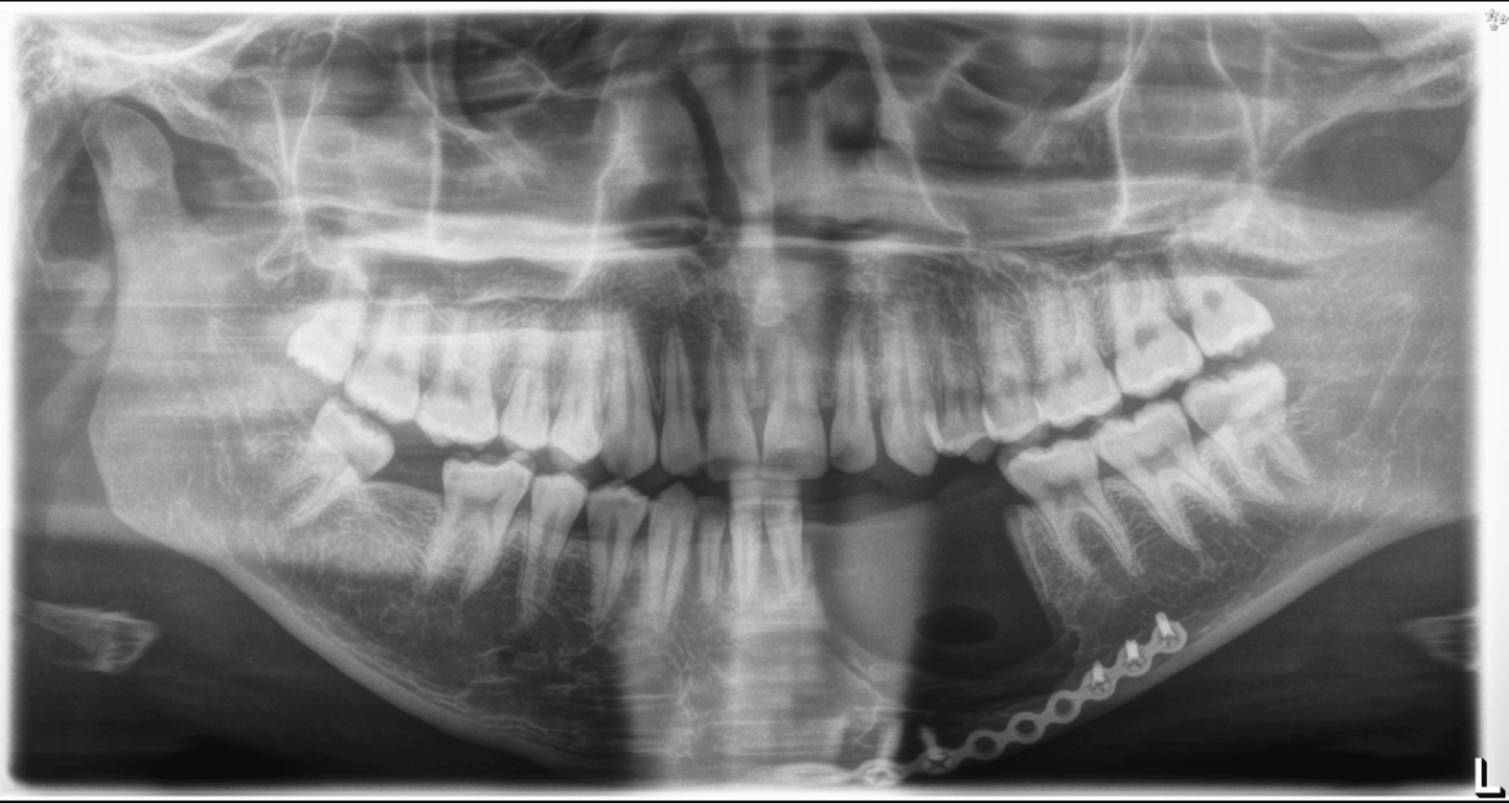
Post-operative OPG at three months. OPG: orthopantomogram
